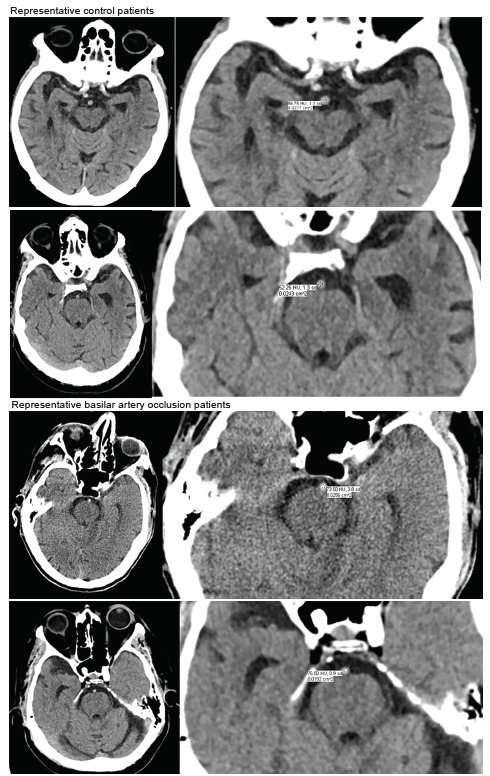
Representative control (above two patients, with full slice CT on left panel and close-up measuring Hounsfield units of basilar artery on right panel) vs basilar artery occlusion (bottom two patients).
